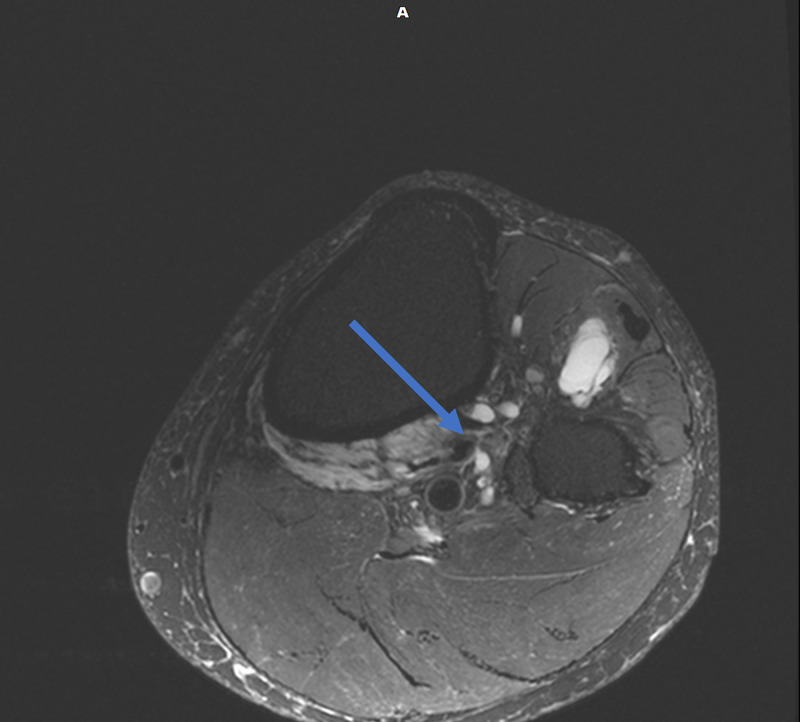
Axial T2-weighted TSE fat-saturated image demonstrating cysts extending to the muscular branch of the popliteus muscle with denervation edema TSE, turbo spin echo
